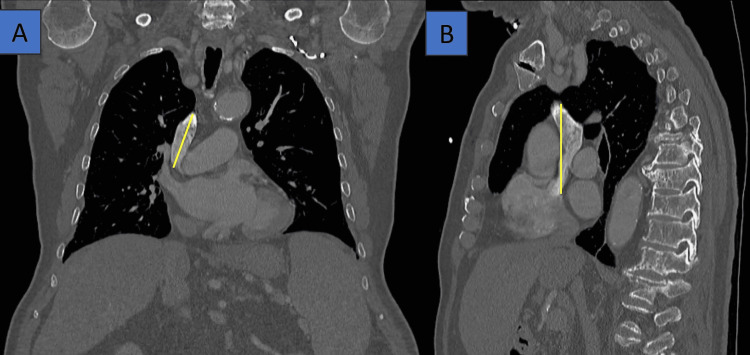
A lateral chest CT with contrast in the bone window. Yellow lines indicate a partially adherent thoracic mural thrombus in the (A) distal arch and (B) descending aorta
